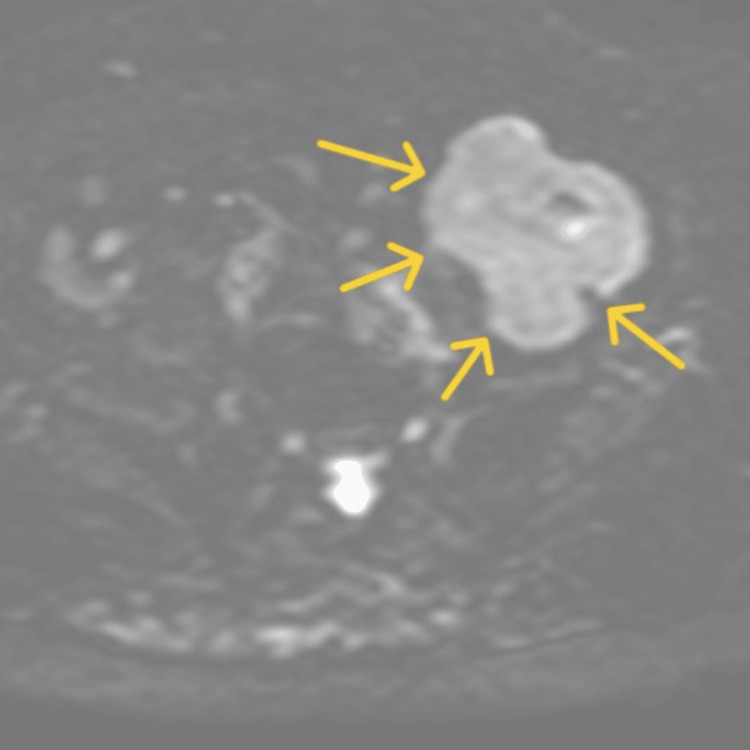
Diffusion-weighted MRI scan. The tumor (yellow arrow) showing high signal intensity on diffusion-weighted imaging, which was suggestive of malignancy.
